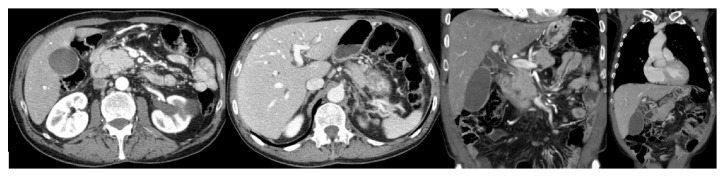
Cervical-thoracic-abdominal-pelvic computed tomography revealed parietal contrast enhancement without intraluminal well-defined mass at the distal common bile duct level with upstream dilatation of the extrahepatic and intrahepatic bile ducts.
